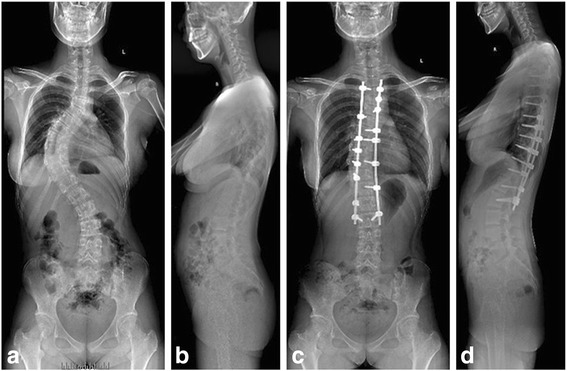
A low-density pedicle screw construct was used. Preoperative standing anteroposterior (a) and lateral radiographs (b). Final follow-up standing anteroposterior (c) and lateral radiographs (d)
